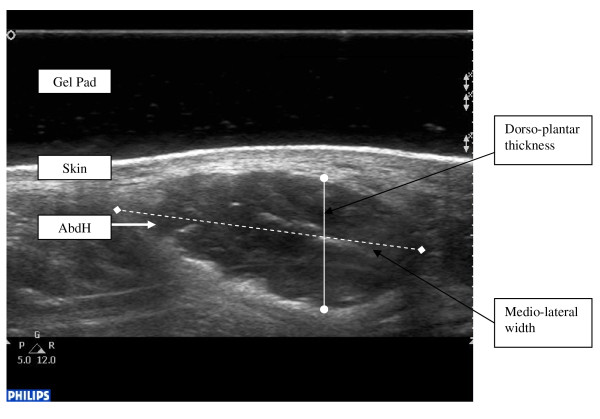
Ultrasound image of abductor hallucis muscle with dorso-plantar thickness and medio-lateral width points marked from Phillips HD11(longitudinal view).
